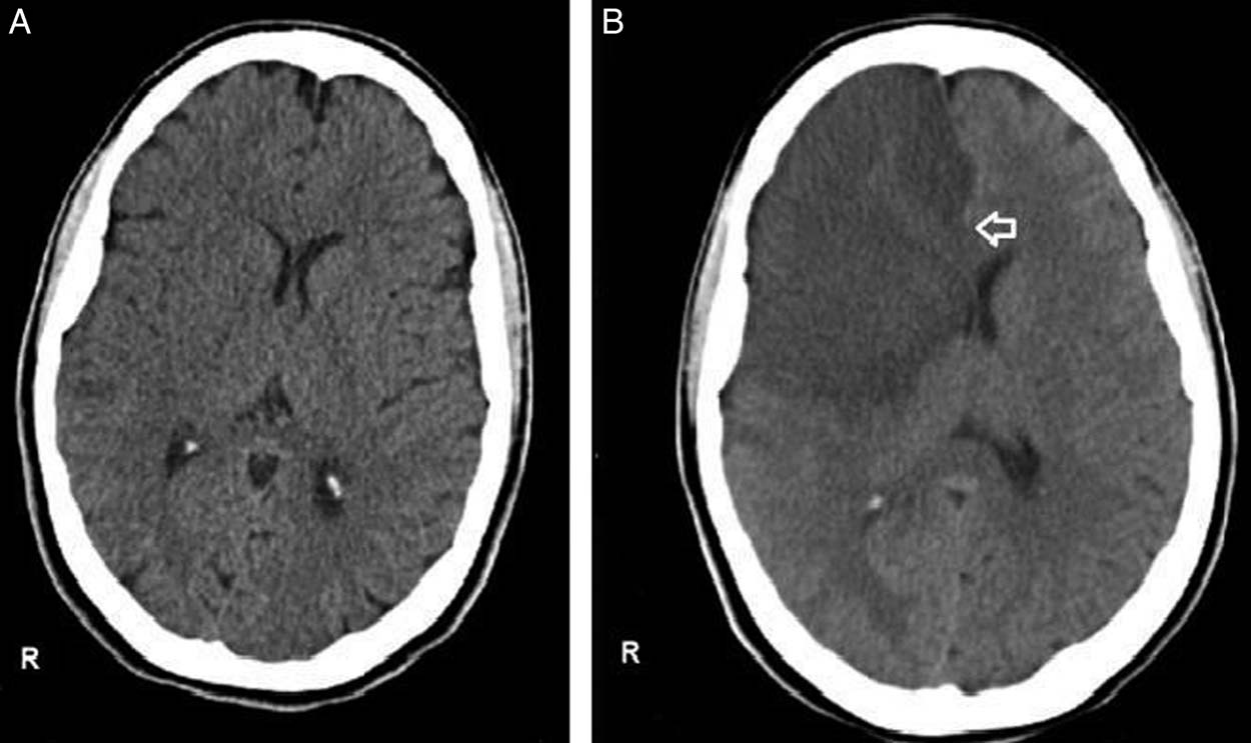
(A) An initial axial head CT scan without contrast upon admission revealed no discernible pathological abnormalities. (B) A subsequent head CT scan without contrast, conducted on the following day, displayed a substantial hypodense area in the right frontal-parieto-temporal lobes, leading to a pronounced mass effect. This was characterized by the loss of distinction between gray and white matter, obliteration of the underlying sulci, compression of the right lateral ventricle, and a substantial 11 mm midline shift toward the left side.
